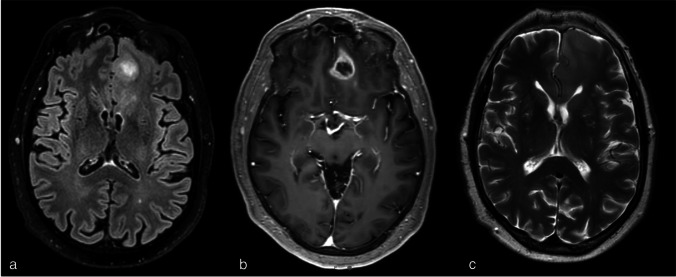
Pre-operative MRI cerebrum: a T2-weighted imaging, b contrast-enhanced T1-weighted imaging, c FLAIR, two (25 mm and 7 mm), rim-enhancing, somewhat erratic delimited lesions left frontal surrounded by an T2/flair hyperintense signal with an edematous aspect of this region, continuing through the genu of the corpus callosum to the right
